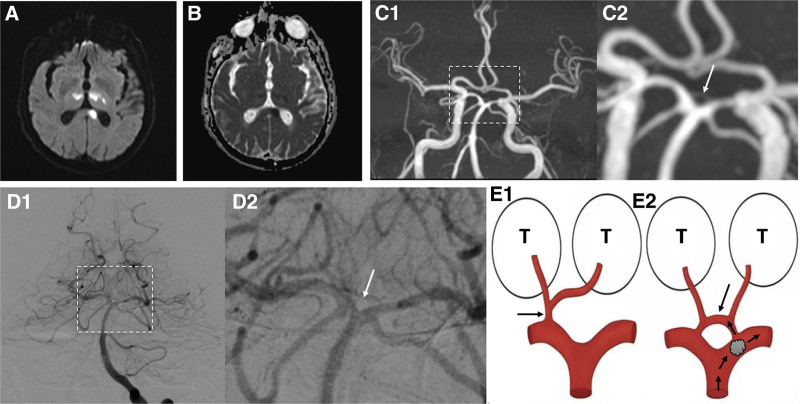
Brain magnetic resonance imaging (MRI) and digital subtraction angiography (DSA). (A and B) Axial diffusion-weighted MRI depicts cerebral infarction in left splenium of corpus callosum and bilateral thalamus with corresponding hypo-intensity in apparent diffusion coefficient sequence. (C and D) MR-angiography and DSA both detect the arcade artery (C1 and D1, magnification shown in C2 and D2 with white arrows). (E) Two uncommon variants (E1, artery of Percheron; E2, the arcade artery and potential stroke etiology in this patient; T = thalamus).
